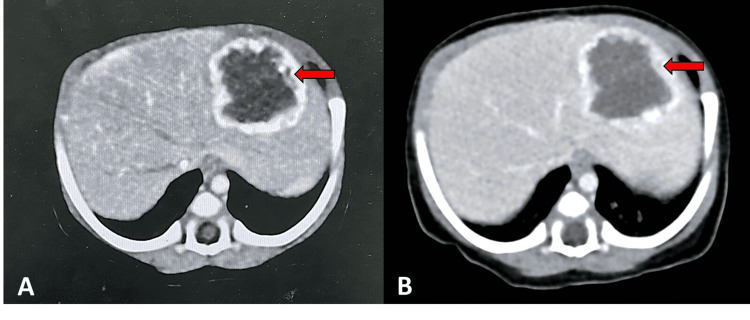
Multidetector CT scan of the upper abdomen using plain CT and dual-phase contrast-enhancement CT. (A) CT in the arterial phase and (B) CT in the portovenous phase demonstrate a hypodense mass with nodular peripheral enhancement in the hepatic segment 2 (red arrow).
